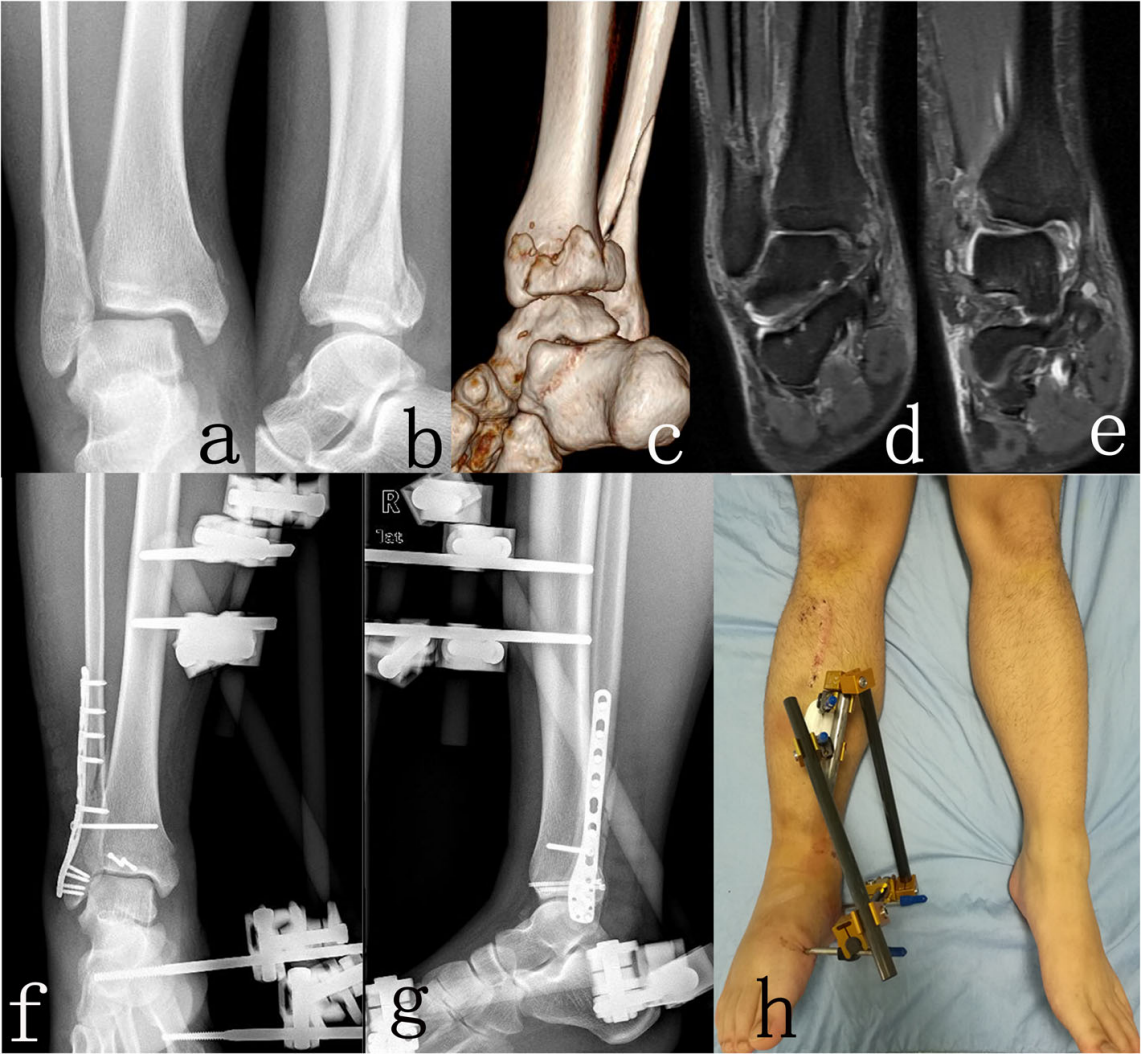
Imaging studies of a 24 years patient with SER IV ankle fractures (AO/OTA classification 44-B3.1) underwent ORIF with TEF. a-c Preoperative X-rays and three-dimensional CT. d, e Preoperative MRI showing deltoid ligament injury. f, g Postoperative anteroposterior and lateral X-rays. h Postoperative appearance of the affected extremity
